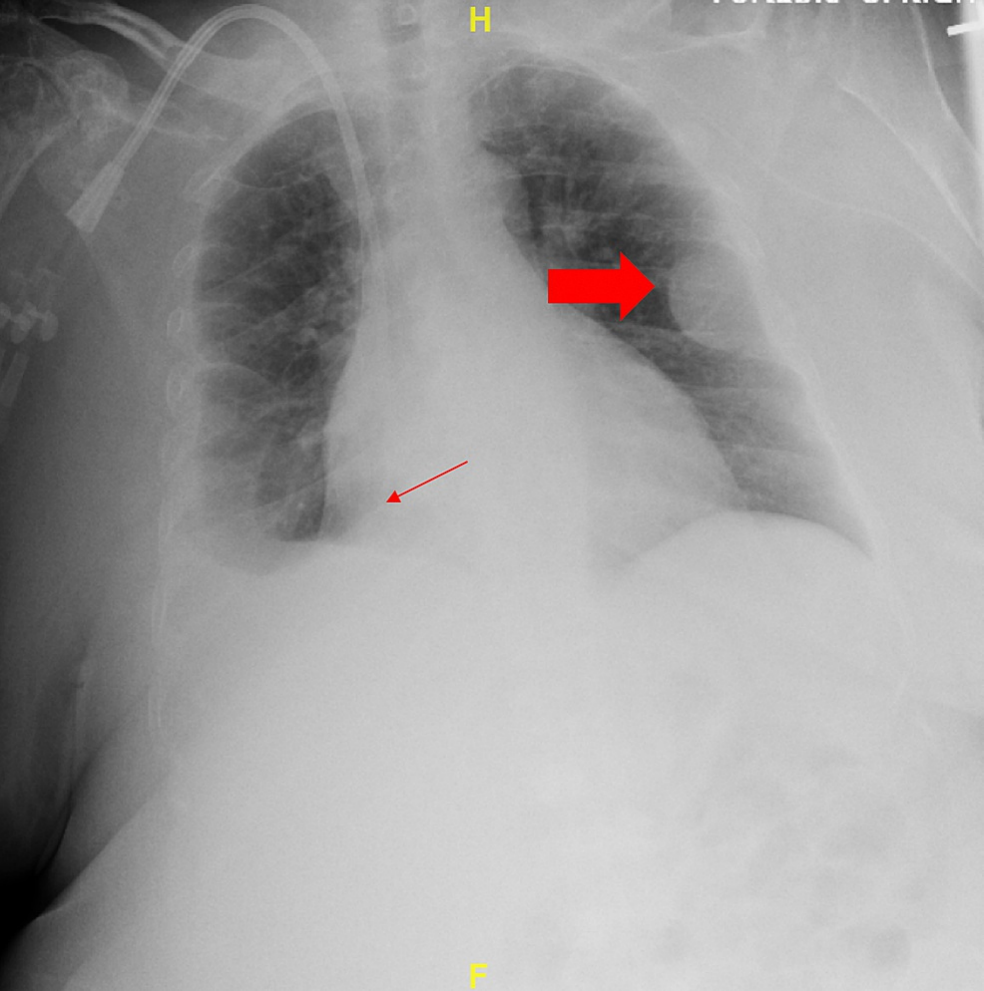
Image showing X-ray of the chest Right-sided pleural effusion (thin arrow) and left-sided pleural-based mass (thick arrow) can be seen.
